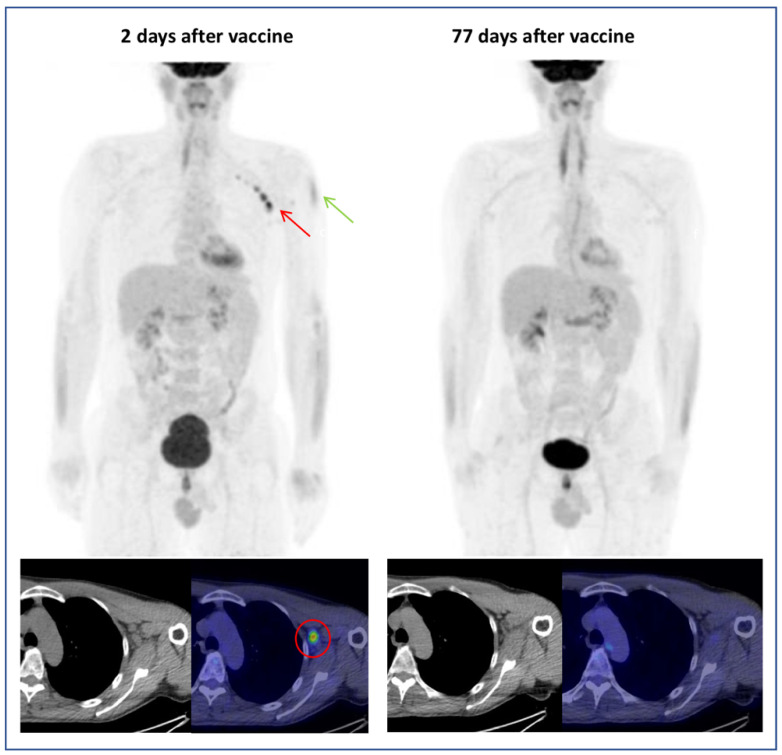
A 53-year-old man in follow-up for left supraclavicular melanoma (pT2aN0M0). [^18^F]FDG PET/CT exam performed 2 days after vaccination showed axillary (SUVmax 9.1) and interpectoral (SUVmax 3.5) hypermetabolic lymphadenopathies (HLNs) (red arrow) and [^18^F]FDG-uptake in left deltoid (injection site, green arrow). Being the HLNs ipsilateral to the site of the previous melanoma, a PET revaluation 77 days after vaccination was performed, showing the disappearance of FDG-avid lymphadenopathies in absence of therapy.
